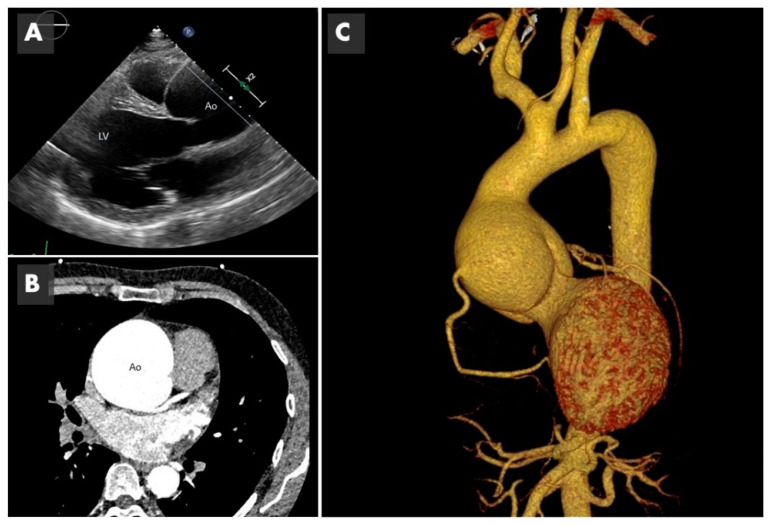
Imaging of bicuspid associated aneurysm. (A) The 2D image of a transthoracic echocardiogram in the parasternal long axis plane showing the dilated aorta (Ao) marked with the straight line (LV: left ventricle). (B) Computed tomography (CT) in axial plane showing the very dilated ascending aorta (Ao) at the level of the sinuses of Valsalva. (C) The 3D CT reconstruction, showing a huge 8 cm aneurysm.
